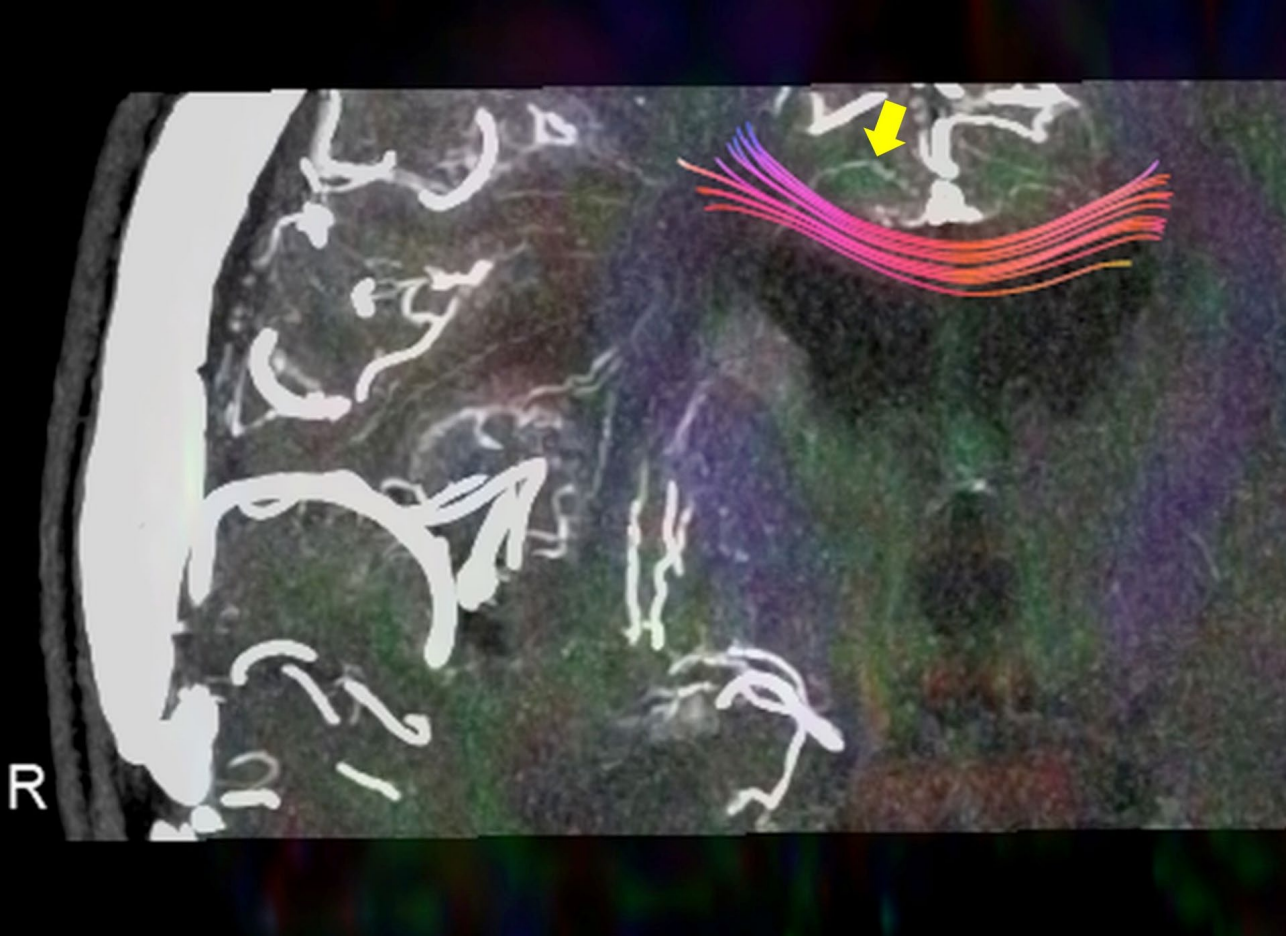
The combination of intra-arterial administration of contrast medium and ultrahigh-resolution computed tomography angiography allowed for observation of the perforating artery supplying the outer part of the corpus callosum running through the cingulate gyrus (arrow). The 3D tractography of the corpus callosum, derived from diffusion tensor imaging, is being fused
